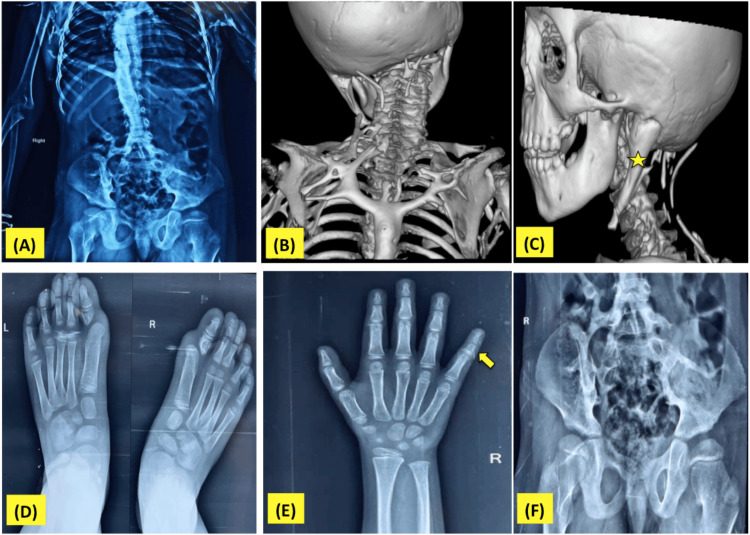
Radiological imaging demonstrating characteristic heterotopic ossification and skeletal anomalies in FOP. (A) X-ray showing a dense sheet-like midline heterotopic ossified band with centrifugal bridging ossifications extending laterally, with pseudo-joint formation with adjacent ribs and iliac bones. (B) VRT image demonstrating extensive heterotopic ossification involving the posterior cervical and upper thoracic paraspinal soft tissues with bridging ossified bands extending towards the scapula. (C) VRT image demonstrating heterotopic ossification along the posterior cervical soft tissues with partial ossification involving the sternocleidomastoid muscle (asterisk), contributing to torticollis and restricted neck movement. (D) Bilateral feet radiographs showing characteristic congenital hallux valgus deformity. (E) Radiograph of the right hand demonstrating shortening of the middle phalanx of the fifth digit (brachymesophalangy) (arrow). (F) Pelvic radiograph revealing broad, short femoral necks bilaterally. FOP: Fibrodysplasia ossificans progressiva; VRT: volume rendering technique
